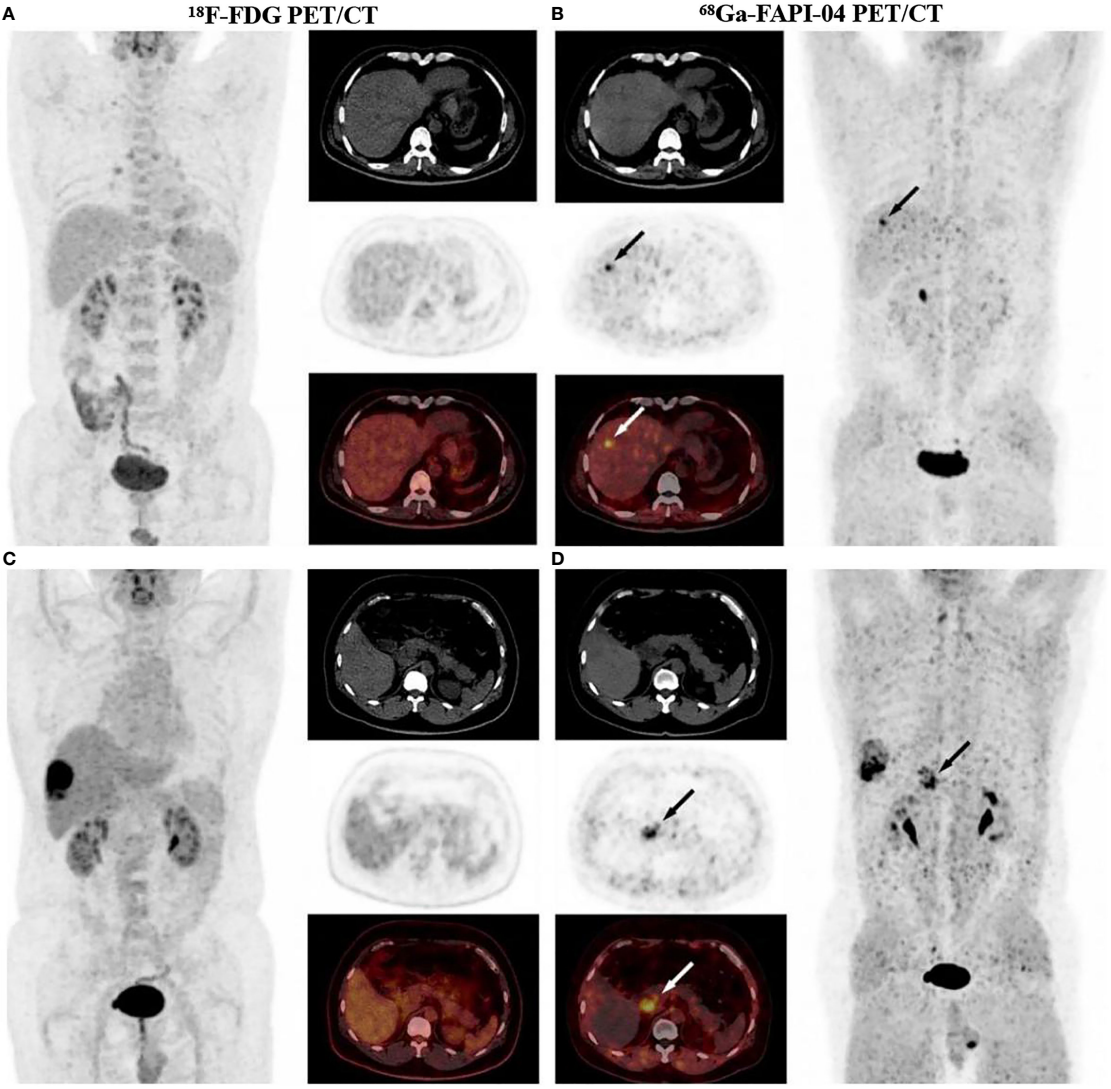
A 53-year-old male diagnosed with moderately-differentiated HCC, ^18^F-FDG PET/CT images did not detect intrahepatic positive findings (A), and ^68^Ga-FAPI-04 PET/CT showed a strongly FAPI positive lesion in the right lobe of the liver (B). In PET/CT images of another 53-year-old male diagnosed I with recurrent HCC and extensive peritoneal diffusion, a small metastatic lesion was not detected in ^18^F-FDG PET/CT (C), which was found with increased uptake in ^68^Ga-FAPI-04 PET/CT (D). Therefore, ^68^Ga-FAPI-04 PET/CT was more sensitive than ^18^F-FDG PET/CT in the detection of HCC and peritoneal metastatic carcinoma. With permission from Wang et al. (45).
